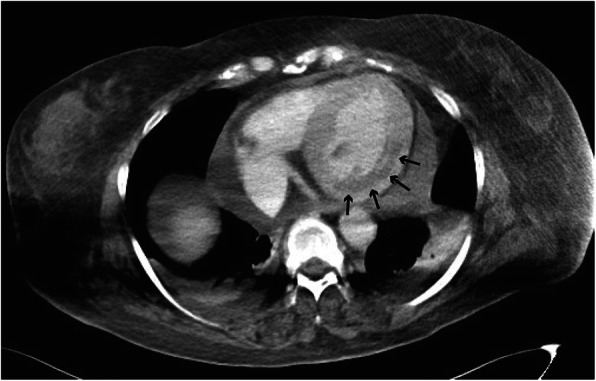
Contrast-enhanced computed tomography. In the contrast-enhanced computed tomography, there is no evidence of pulmonary embolism. However, a contrast-opaque area on the intimal side of the left ventricular inferior wall can be seen (indicated by the black arrows)
